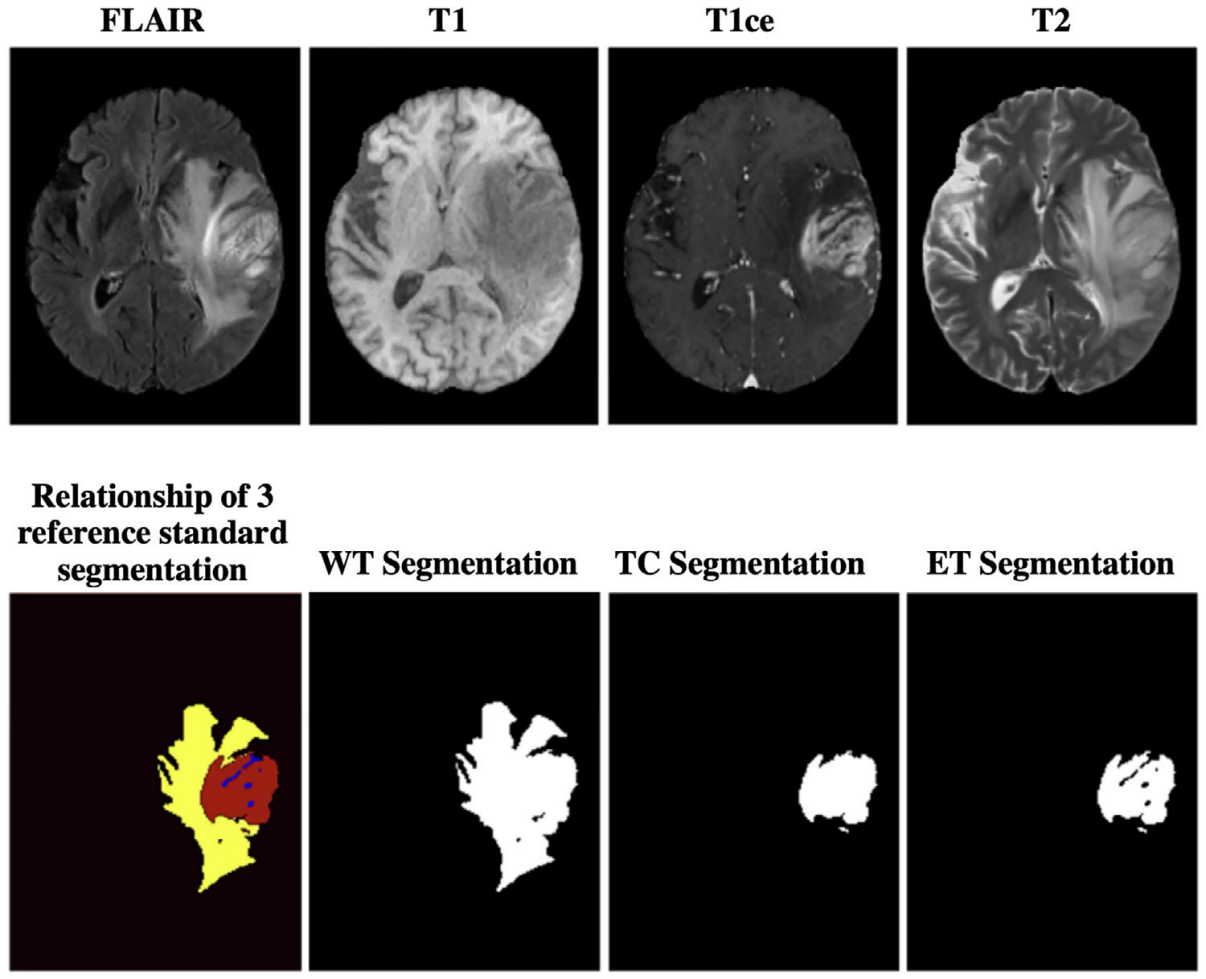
An example of 4 MR images (i.e., FLAIR, T1, T1ce, and T2) and the corresponding 3 reference standard segmentations (i.e., ET, TC, and WT) from the BraTS challenge 2020 dataset.
